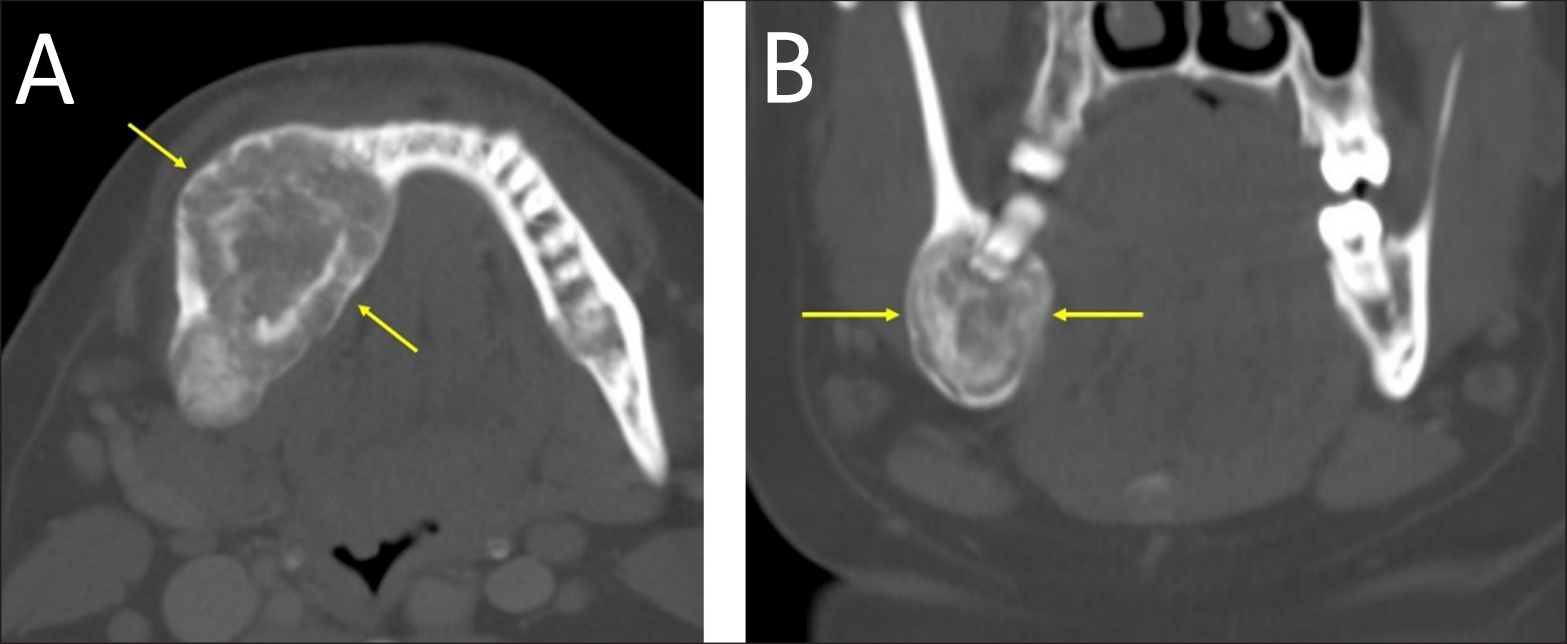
(A, B) Cemento-ossifying fibroma. Axial and coronal CT images showing and expansile lesion with sclerotic internal components (arrows).
